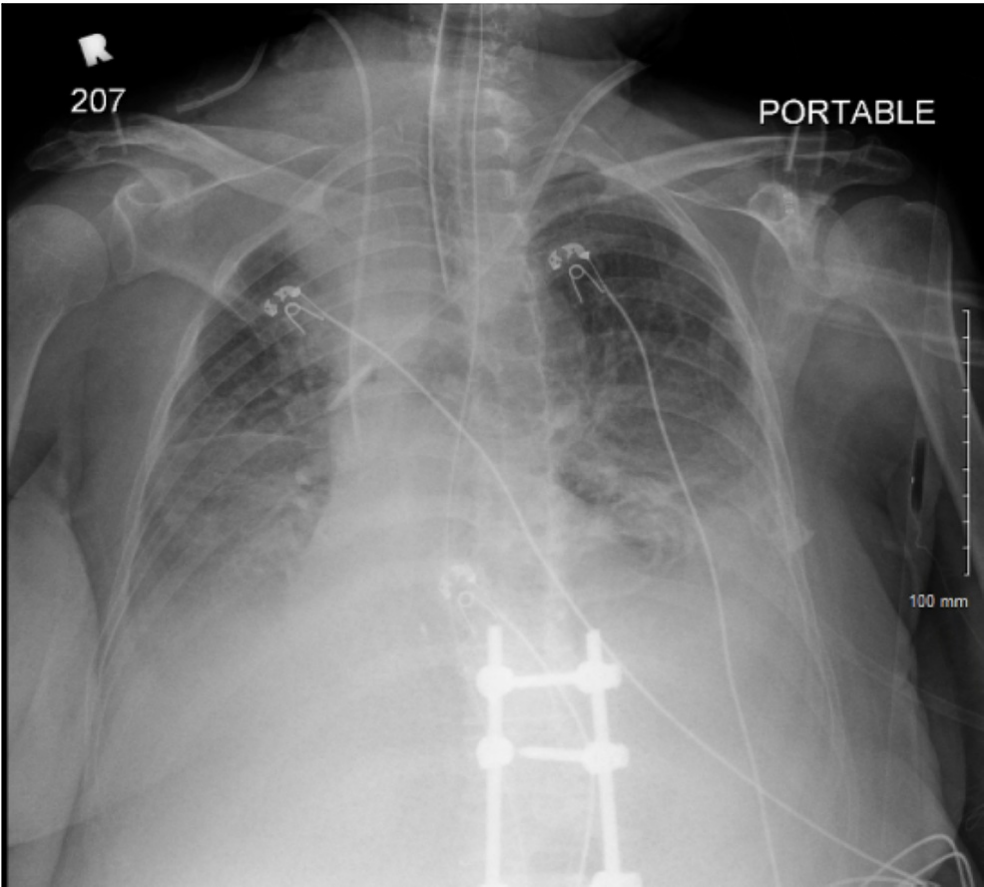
Final Chest X-Ray two days prior to expiration.
